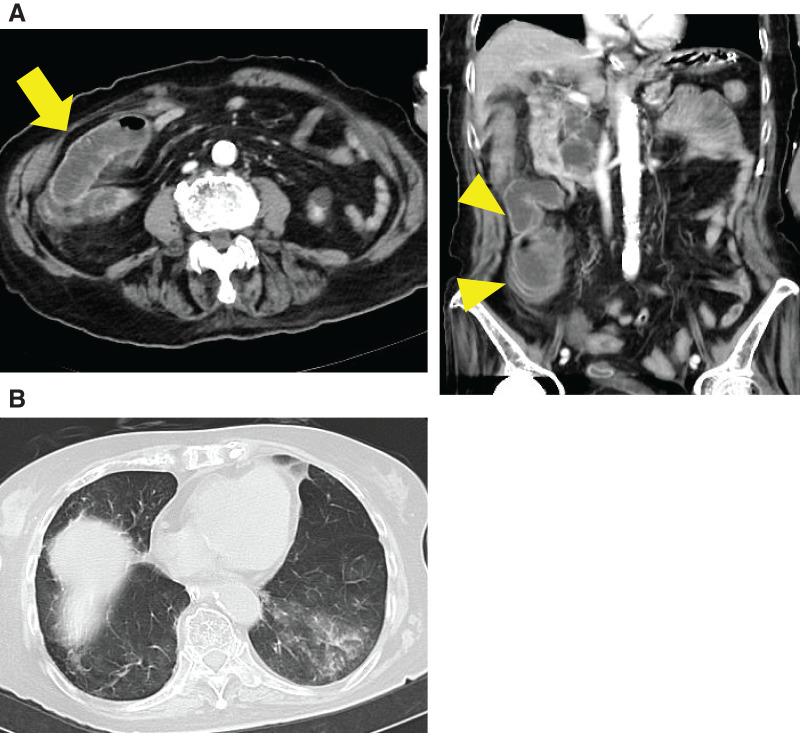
Preoperative imaging findings. (A) Abdominal CT scan shows dilated small bowel loops and a closed-loop obstruction (arrow, left panel). Coronal CT image highlights the herniated small bowel (arrowhead, right panel). (B) Chest CT demonstrates ground-glass opacity in left lung fields, suggesting aspiration pneumonia.
